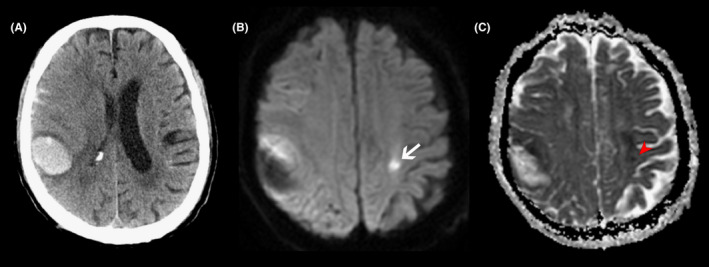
Representative MRI of diffusion‐weighted imaging lesions (DWILs) in patients with acute intracerebral hemorrhage. In a patient with lobar hemorrhage (A), the lesion (white arrow) appears as hyperintensity on diffusion‐weighted imaging (B) with corresponding hypointensity signal (red arrow) on apparent diffusion coefficient map (C).
